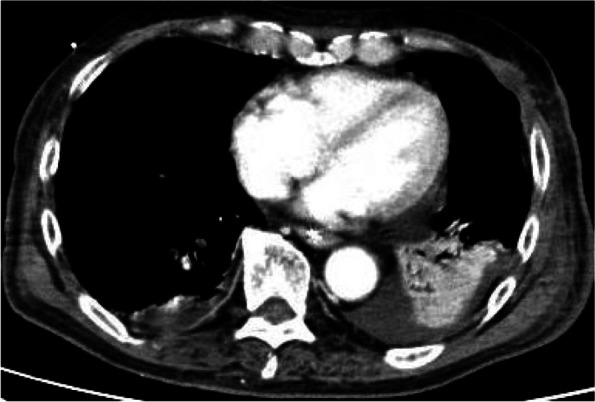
Chest computed tomography scans showing atelectasis in the left lower lung field on day 9, before intrapulmonary percussive ventilation therapy
